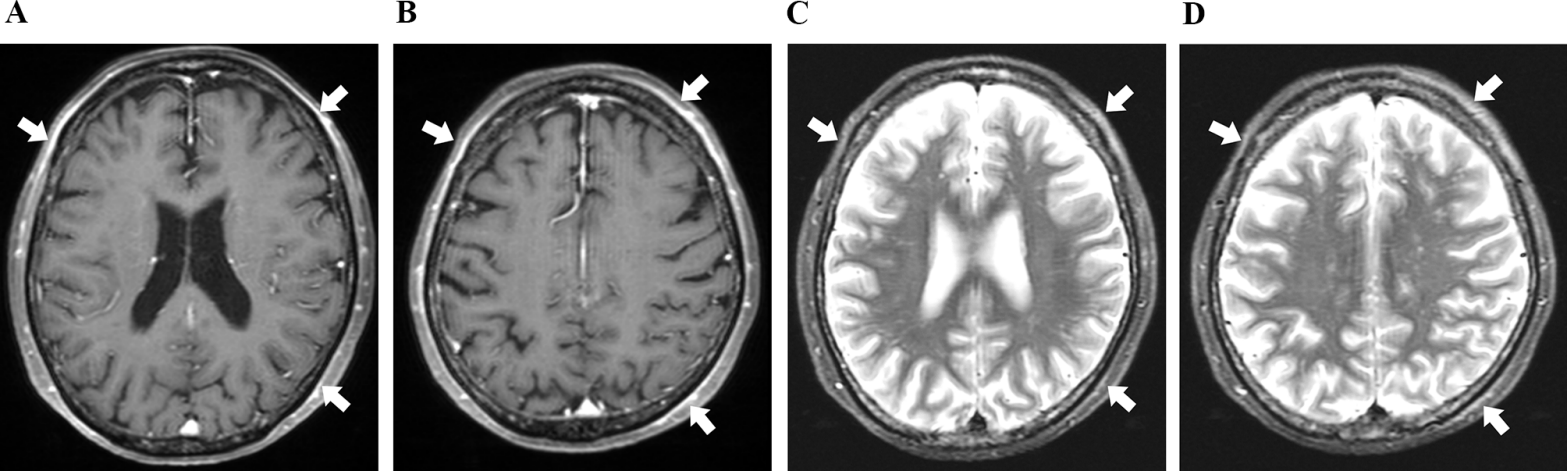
3 Case 8 (giant cell arteritis). A and B, Contrast-enhanced T1-weighted (CE-T1W) images show multiple distant arcuate enhancement areas of extracranial soft tissues. C and D, STIR images show multiple distant high signal intensity areas of extracranial soft tissues
